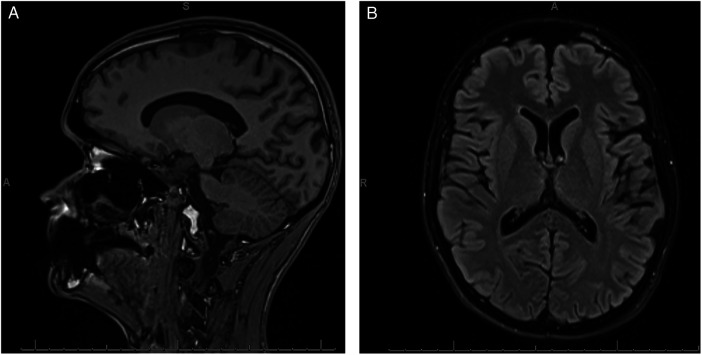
(A,B) Axial and sagittal T1 MPRAGE MRI of the patient’s head. The image was taken 14-month postsurgical intervention when the OCD was subclinical (youth report).
